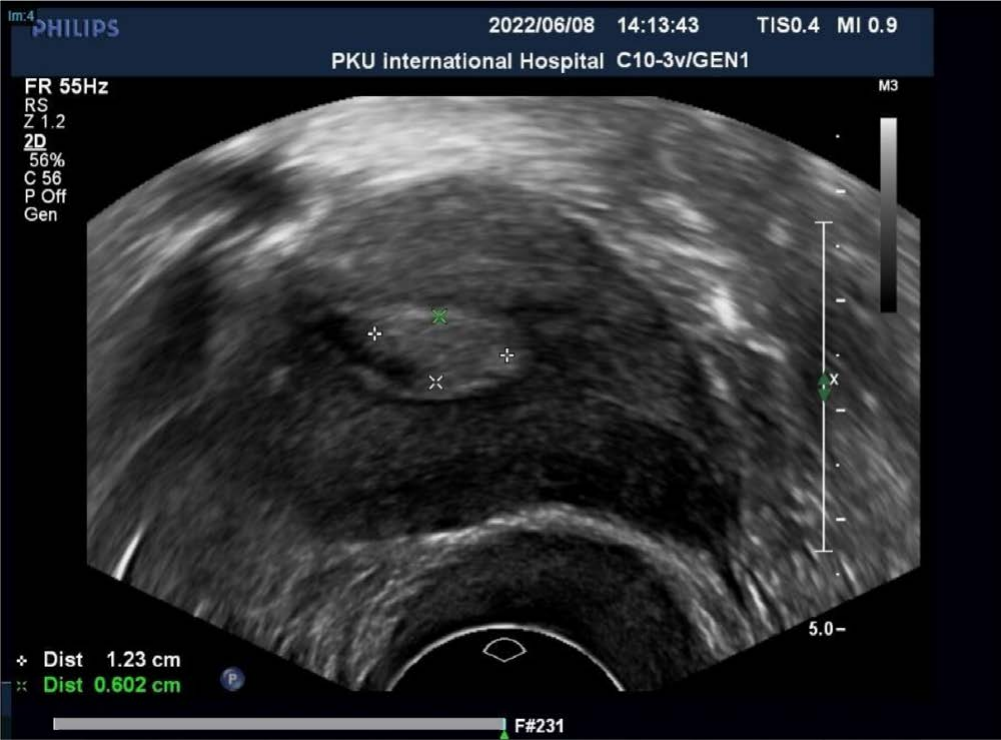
Transvaginal ultrasonography revealed a space-occupying lesion measuring 12.3 × 6 mm in the uterine cavity.
